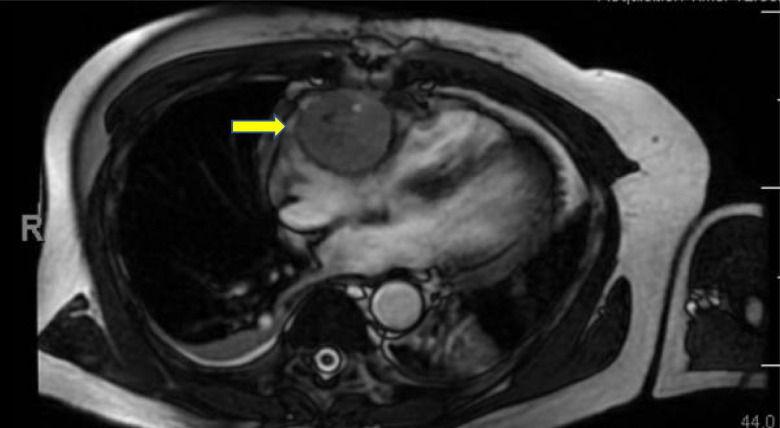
Cardiac MRI: 6.9 × 5.8 × 4.9 cm mass involving the right lateral wall, atrioventricular groove, and encasing the right coronary artery.
